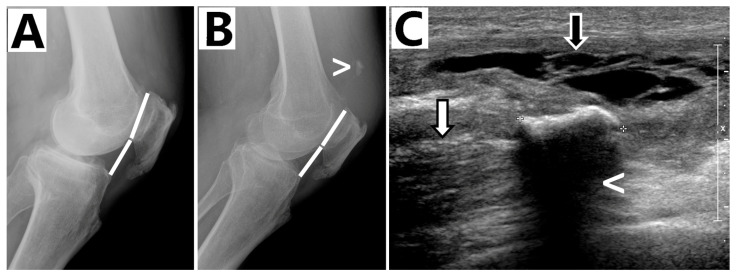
(A) Caton–Deschamps Index values at trauma of 0.8; (B) Caton–Deschamps Index values at pre-op of 0.73. Enthesophyte of the quadriceps tendon (>); (C) Ultrasound scan (Pre-operative): Partially organized hematoma with some echogenic septa inside (black arrow); entesophyte (<) of the quadriceps tendon avulsed from the upper pole of the patella and retracted proximally (white arrow).
